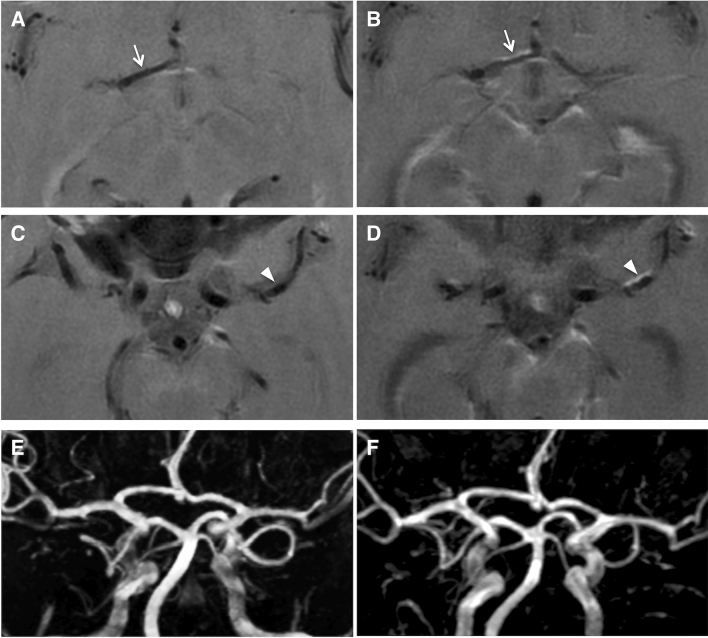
Progressive vessel wall imaging findings on follow-up in a patient with CNS vasculitis due to cryopyrin-associated periodic syndrome. Follow-up vessel wall imaging performed 34 months after the initial presentation (B, D) depicts contrast enhancement along the anterior vessel walls of the right A1 segment (arrow) and the left M1 segment (arrowhead), which was not identifiable on the initial MRI scan (A, C). Perivascular contrast enhancement surrounding the posterior cerebral arteries can be seen on both scans. Correlating TOF-MRA images (E, F) at both times do not show stenoses of the arteries of the circle of Willis (“MRA-negative”). The patient was under immunosuppressive therapy for the follow-up period
